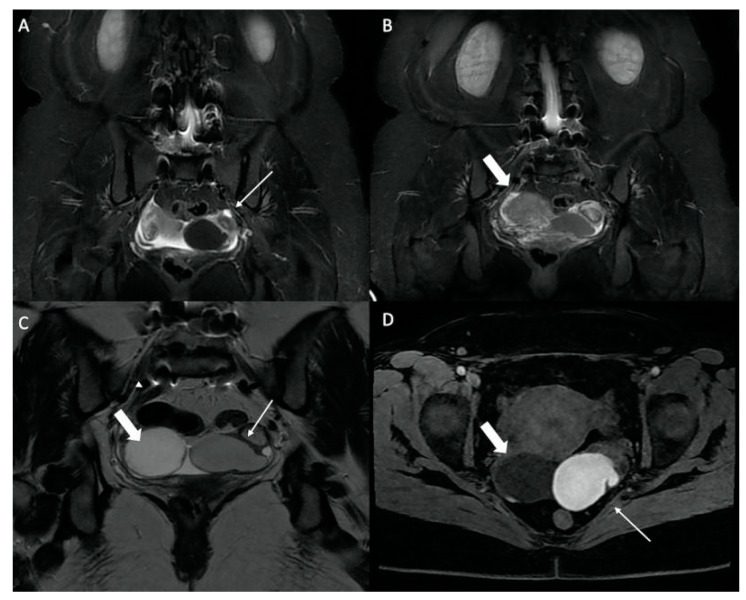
Assessment of bilateral sciatica in a 44-year-old woman. (A) Coronal STIR MRI. Left ovarian lesion in homogeneous STIR hyposignal (small arrow) with low abundance pelvic fluid effusion. (B) Coronal STIR MRI. Right adnexal lesion with heterogeneous signal (thick arrow). (C) T2 coronal MRI and (D) T1 axial MRI with saturation of the fat signal (complementary remote assessment). Right ovarian cystic lesion in T1 hyposignal, homogeneous T2 hypersignal, with small dependent hemorrhagic contingent in T1 hypersignal (thick arrows). Left ovarian lesion with “dirty” intermediate T2 signal with T2 shading sign, in spontaneous T1 hypersignal (small arrows). Aspect of “kissing ovaries” with ovarian adhesion in retro-uterine position. Left endometrioma and posterior deep pelvic endometriosis. Hemorrhagic right ovarian cyst.
